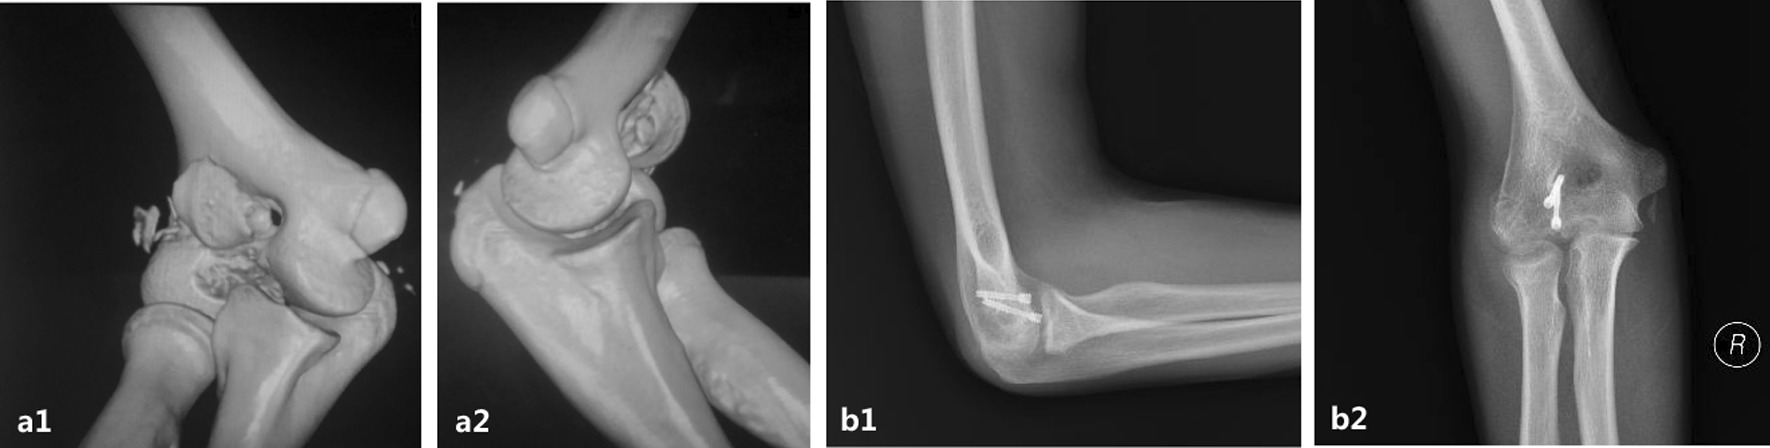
A 16-year-old boy diagnosed with AO type 13B3.2 humeral trochlear fracture (a1, a2). At the final follow-up, plain roentgenograms showed good bone union with good function (b1, b2)
